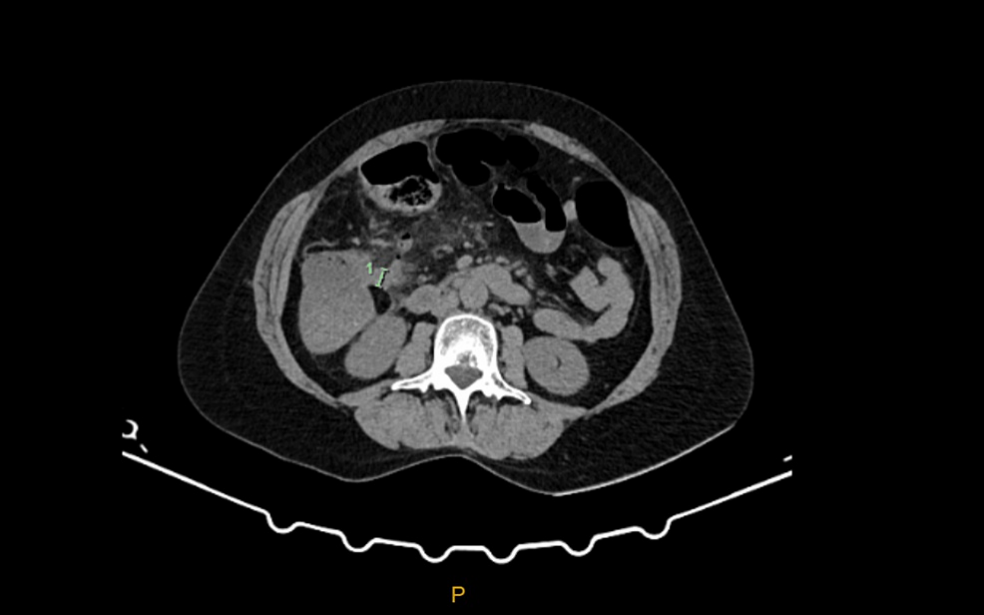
CT scan axial view showing the base of the cecum and appendix with surrounding inflammation.
